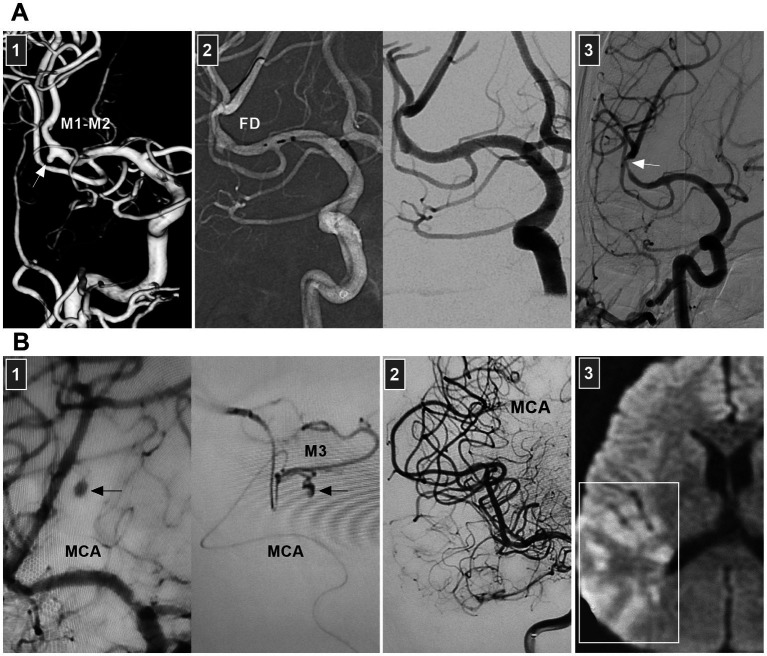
Complication from EVT for MCA dissections. (A) Panel 1: DSA showing a sidewall dissection at M1–M2 junction (arrow). Panel 2: Roadmap image (left panel) and DSA (right panel) showing the dissection was covered by FD. Panel 3: Six-month follow-up DSA showing that the dissection was cured and there was a stenosis of M2 (arrow). (B) Panel 1: DSA (left panel) and microcatheter angiography (right panel) showing a small dissection of M3 (arrows). Panel 2: DSA showing the dissection was embolized by casting liquid embolic agent. Panel 3: Postoperative magnetic resonance image showing that the acute infarction of parietal lobe (frame). DSA, digital subtraction angiography; EVT, endovascular treatment; FD, flow diverter; MCA, middle cerebral artery; M1, first segment of MCA; M2, second segment of MCA; M3, third segment of MCA.
